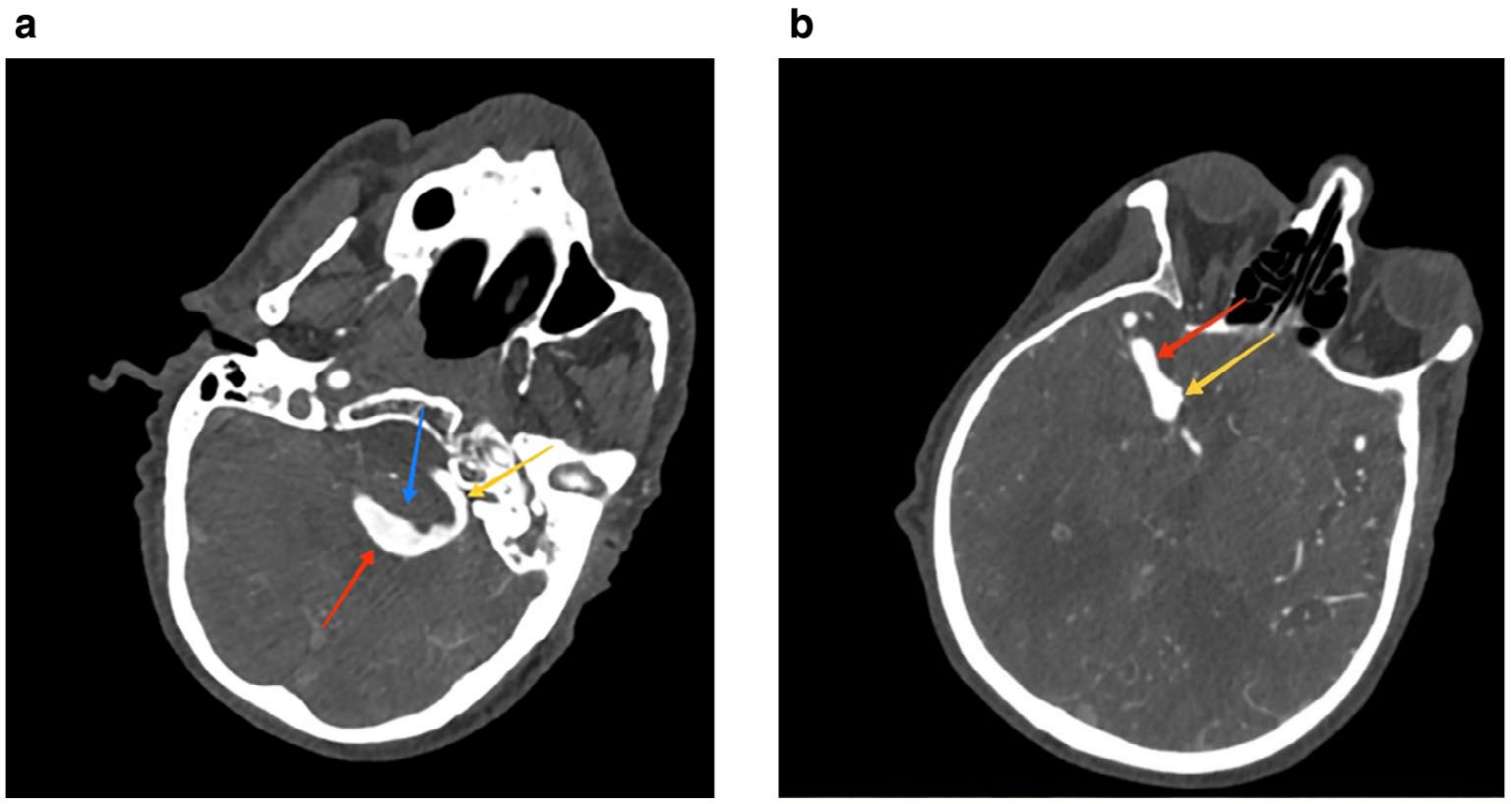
(a) CT cerebral angiography axial section at the level of 4th ventricle showing contrast opacification of residual lumen (red arrow) of partially thrombosed (blue arrow) fusiform aneurysm V4 segment of left vertebral artery. Lumen of aneurysm is continuous with left vertebral artery proximally (yellow arrow). (b) CT cerebral angiography axial section at the level of lateral ventricles showing ectatic terminal internal carotid artery (yellow arrow) and M1 segment of right middle cerebral artery (red arrow).
